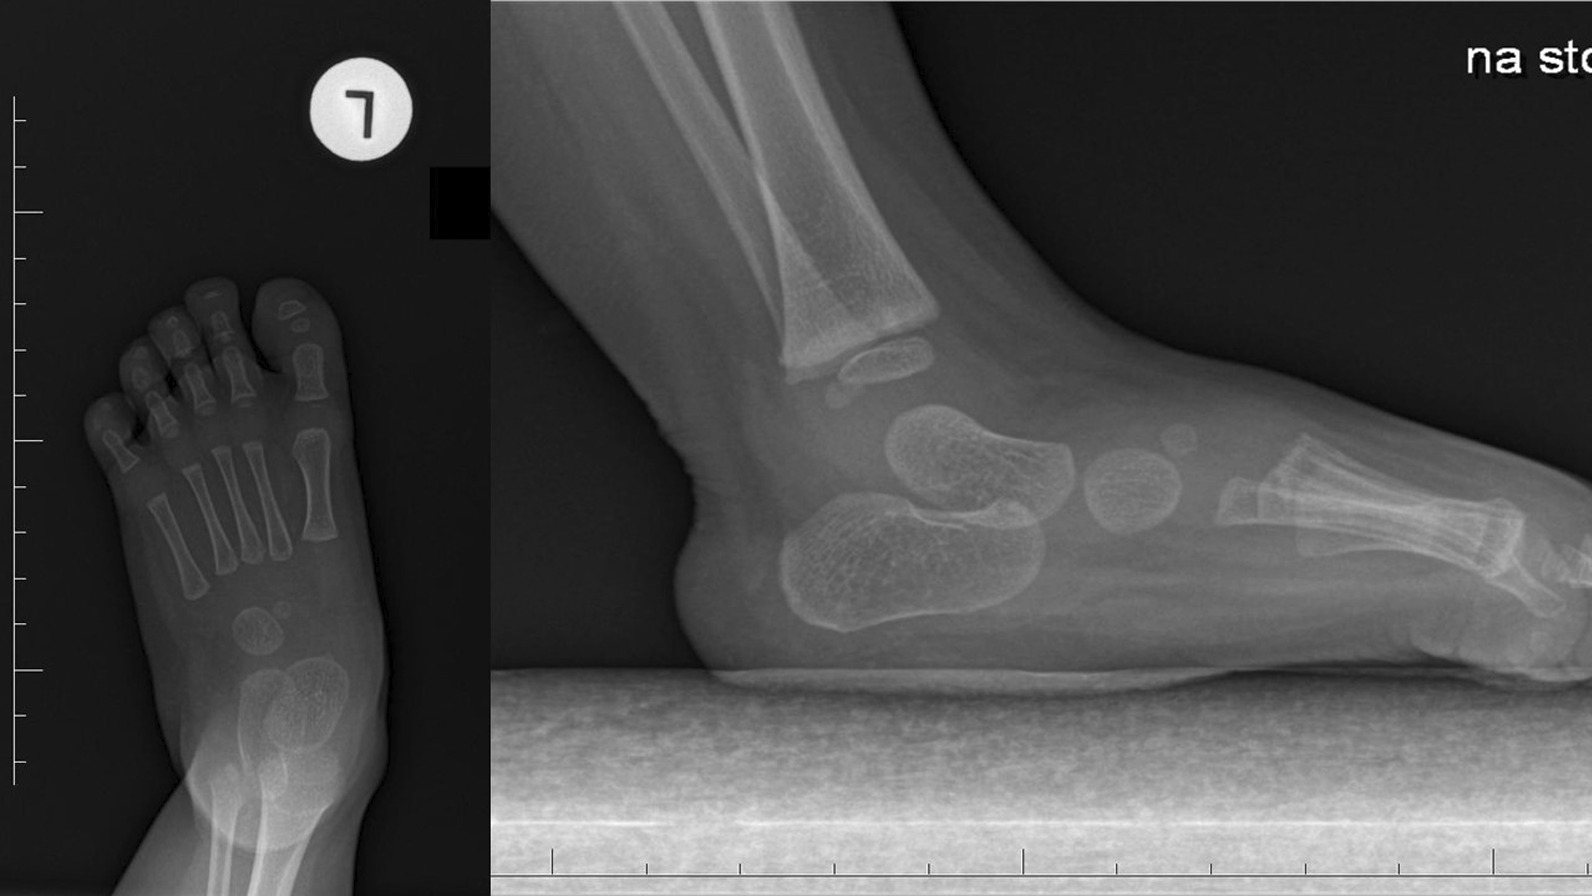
Case 1 AP and L X-ray 6 months after surgery
